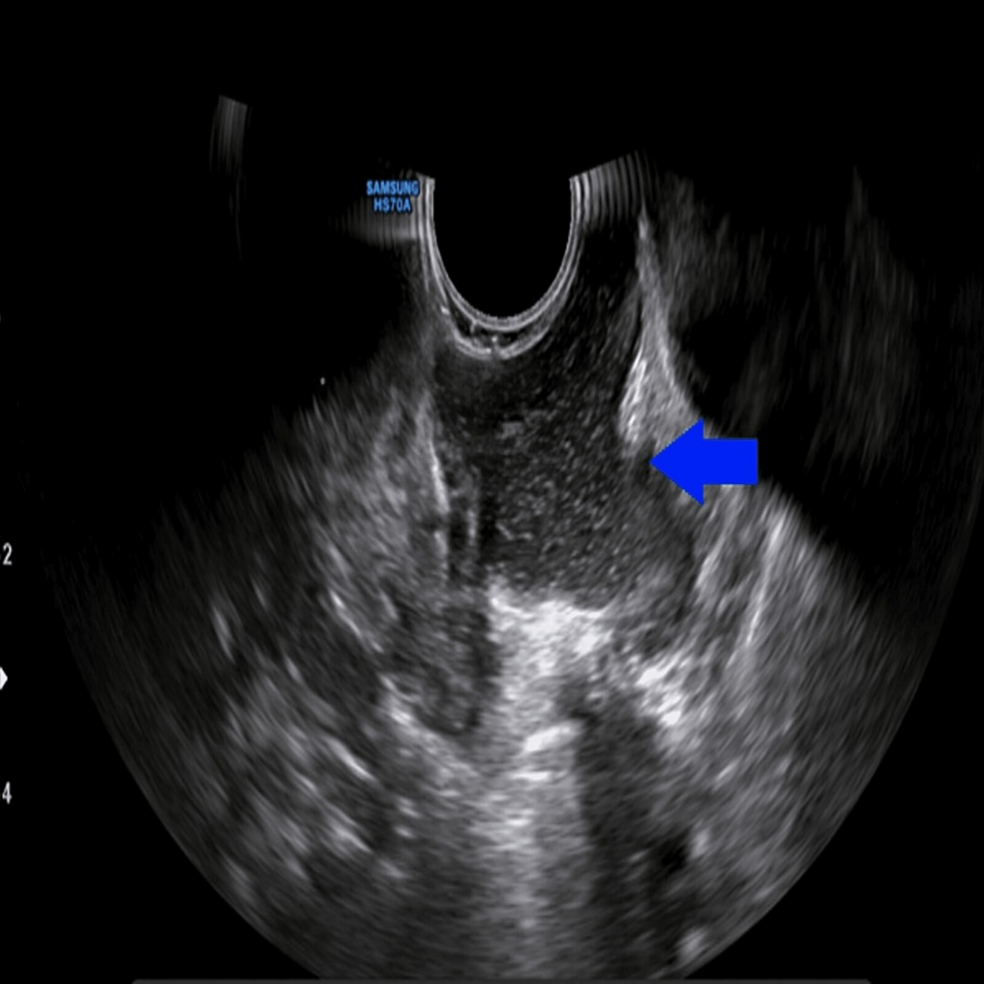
Intraoral B-mode ultrasound showing a sublingual component as a well-defined cystic lesion in the floor of the mouth with multiple internal echoes within it (blue arrow).
